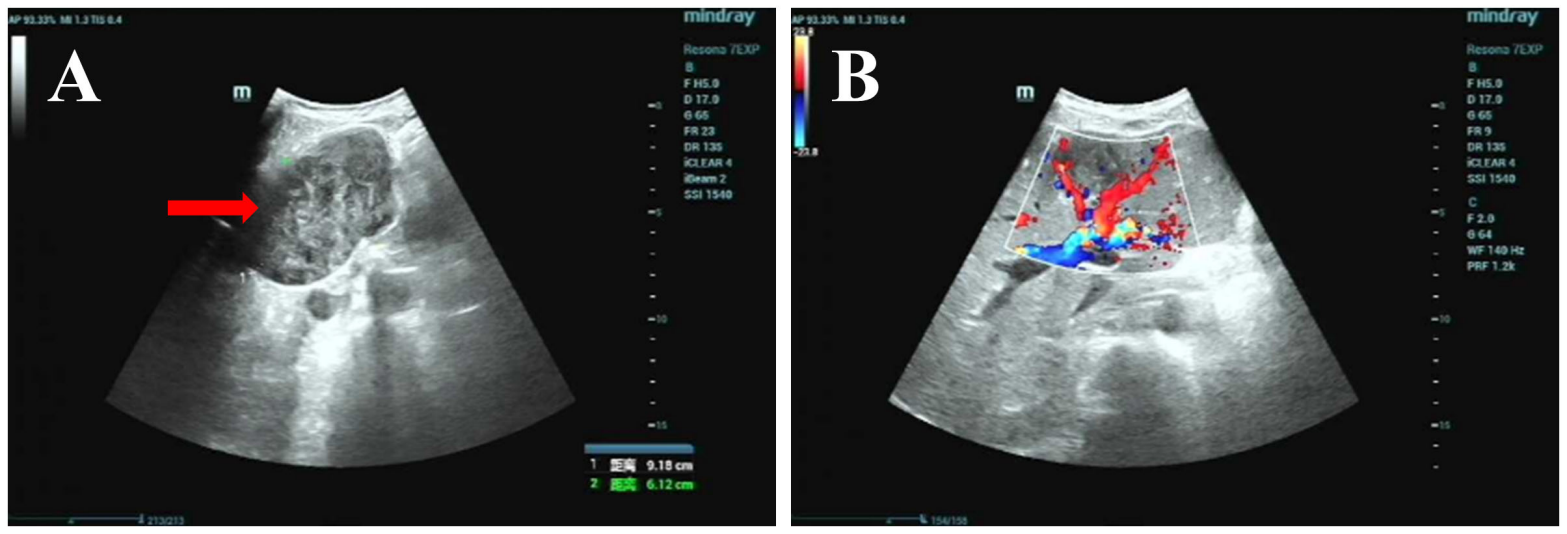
Ultrasound image: (A) Grayscale ultrasound of the left hepatic lobe, showing a 9.2×6.1 cm heterogeneous echoic mass with clear borders and irregular shape (red arrow); (B) Color Doppler ultrasound of the same region, showing vascular filling (red/blue signals) in surrounding tissues and no abnormal intratumoral blood flow (red arrow).
